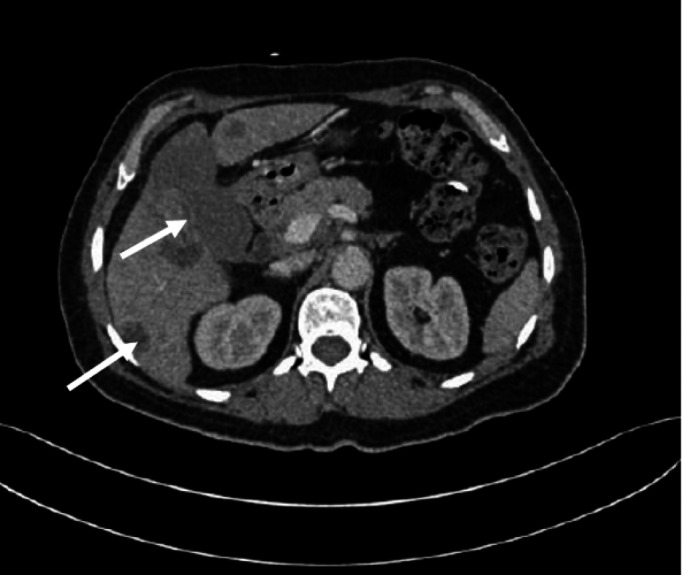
Abdominal computed tomography showed partial response after two cycles of chemotherapy.
